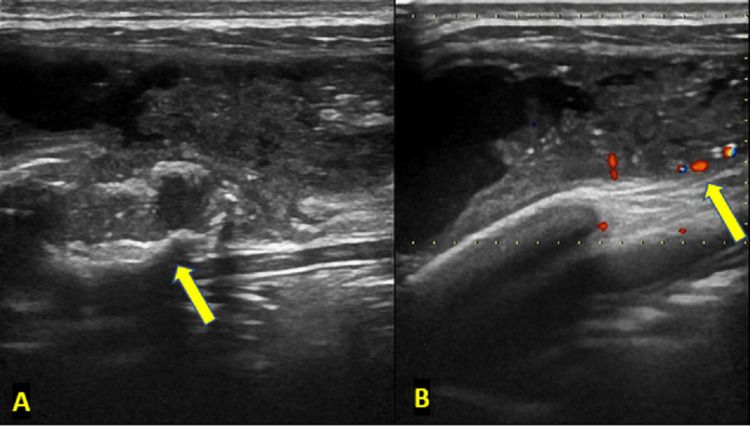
(A) B-mode gray scale ultrasound image showing rib erosion (depicted by arrow) with adjoining abscess cavity seen anterior to it. (B) Color Doppler image of the abscess showing minimal peripheral vascularity (depicted by arrow).
